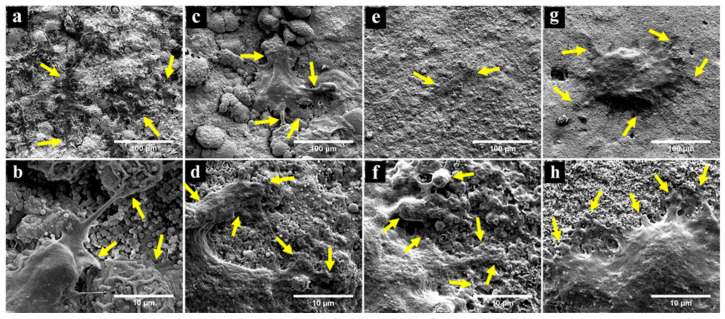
FESEM observation showing successful attachment and spread of SHED on (a,b) APC, (c,d) APC-0.6%CT, (e,f) APC-1.25%CT and (g,h) APC-2.5%CT after 3 days. Yellow arrows in upper images (a,c,e,g) focus on cell attachment and extension of cytoplasmic processes. Yellow arrows in high-magnification images (b,d,f,h) point to the interface between lamellipodial and filopodial protrusions and the material surface. Magnification of 1000× (upper) and 10,000× (lower).
